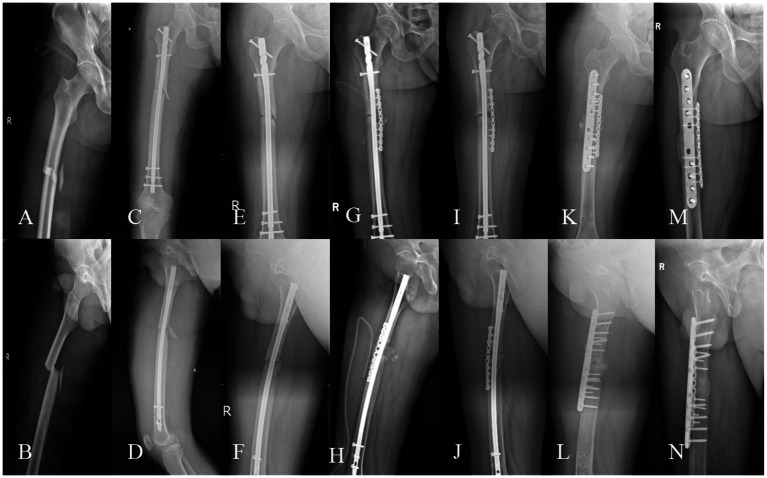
A 39-year-old male, BMI: 22.16 kg/m^2^, non-smoker, no metabolic diseases. (A,B) Right femoral shaft fracture sustained in a car accident on January 5, 2021. (C,D) Closed reduction and intramedullary nailing performed on January 13, 2021. (E,F) Nonunion with minimal callus formation observed during follow-up on October 8, 2021. (G,H) Revision surgery on October 23, 2021, retaining the original nail and augmenting with autologous iliac cancellous bone grafting and an auxiliary plate. (I,J) Persistent nonunion noted during follow-up on July 7, 2022. (K,L) Second revision surgery on July 12, 2022, utilizing dual locking plate fixation, PRP-augmented structural iliac bone grafting, and bioactive core construction. (M,N) Excellent radiographic union observed at 6.2 months postoperatively, with a lower extremity functional score of 65 and a VAS pain score of 1.
